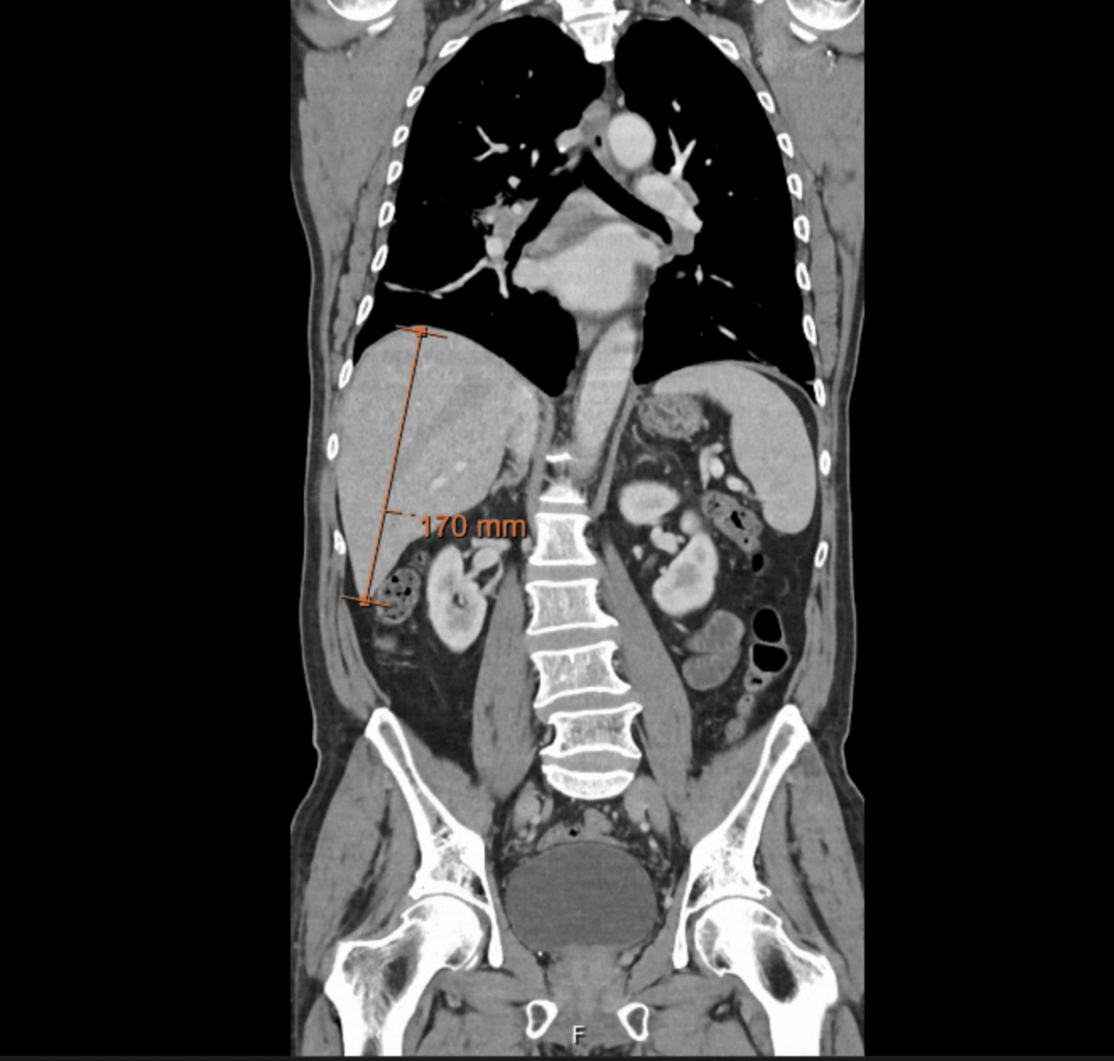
Coronal abdominopelvic computed tomography (CT) demonstrating hepatomegaly, with the right hepatic lobe measuring 17 cm (arrow).
